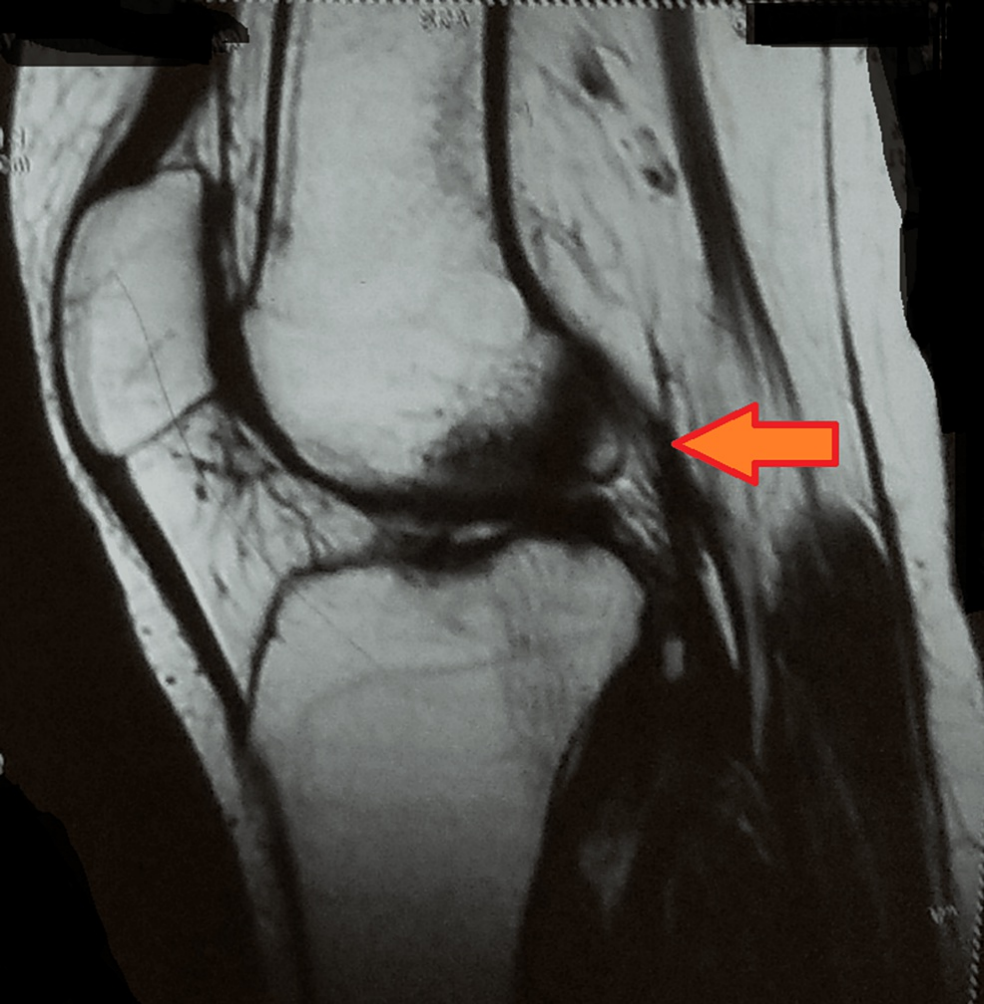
Magnetic resonance imaging- left knee joint Arrow showing hyperintensity in the lateral condyle of the femur and tibia
